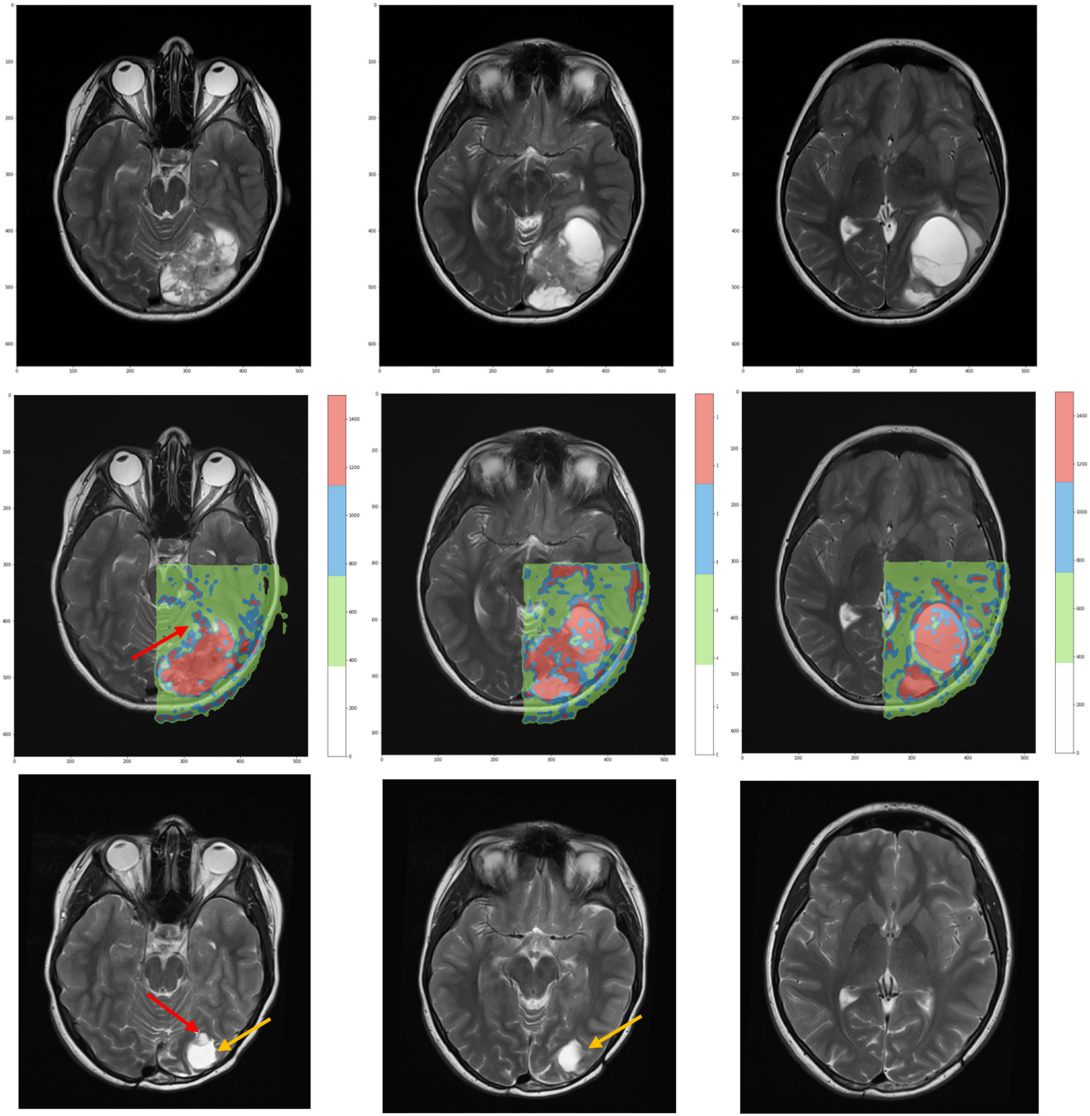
(Top) T2-weighted MRI acquisitions showing anatomical details of tumor at time of diagnosis. (Center) Overlay of the network classification results, with the red subnetwork covering the tumor and some other external areas. The cluster subnetwork depicted in green identifies the surrounded brain. Finally, the blue bridges linking together the two subnetworks surround the red regions and act as interface between tumor and brain. (Bottom) T2-weighted MRI acquired 3 years after surgical and proton therapy treatment highlights the presence of recurrence (red arrow at leftmost plot) and post-surgery cavity (tumor bed, orange arrow at both leftmost and central plots).
